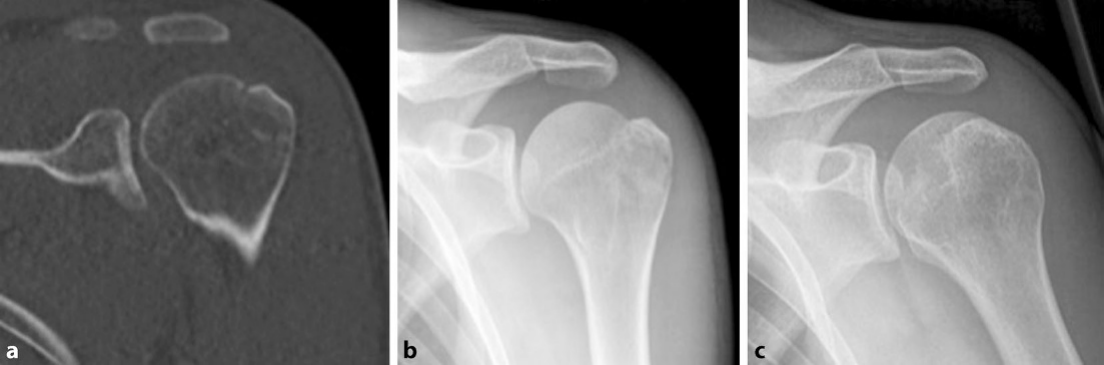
Conservatively treated fracture of the greater tuberosity: initial radiograph (a), follow-up after 3 weeks to exclude secondary displacement (b), and anatomic consolidation 3 months after the injury (c)
